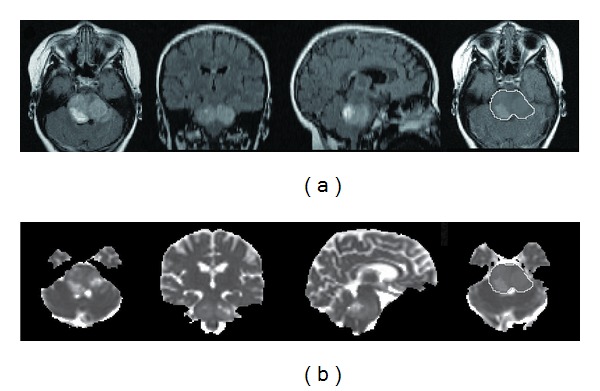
Coregistered axial, coronal, and sagittal (a) FLAIR images and (b) MD maps of the pons. ROIs were outlined on axial images ((a) and (b) far right) and combined to create a volumetric ROI covering the entire lesion.
